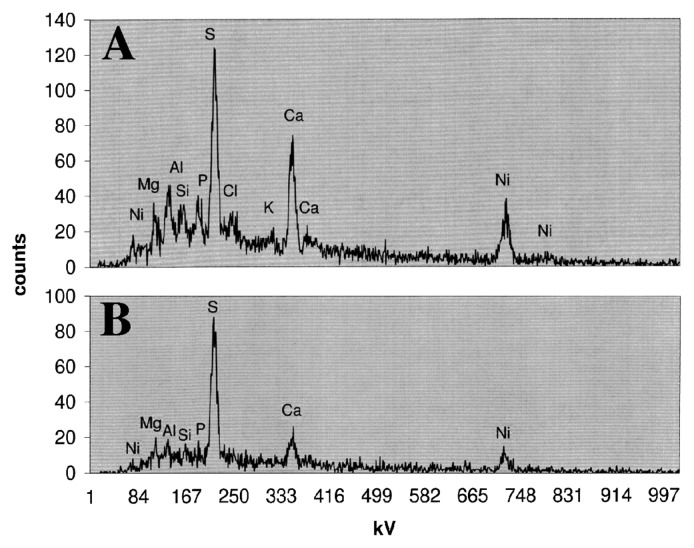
X-ray microprobe scans of microcolonies (A) and general biofilms excluding microcolonies (B) treated with 10,000 μg L^−1^ nickel. The detection minimum for this analysis was 2,000 μg L^−1^ w/w in dried biofilm material. Since peak heights were proportional to concentrations, the microcolony material had approximately 4-fold higher levels of nickel than general biofilms.
